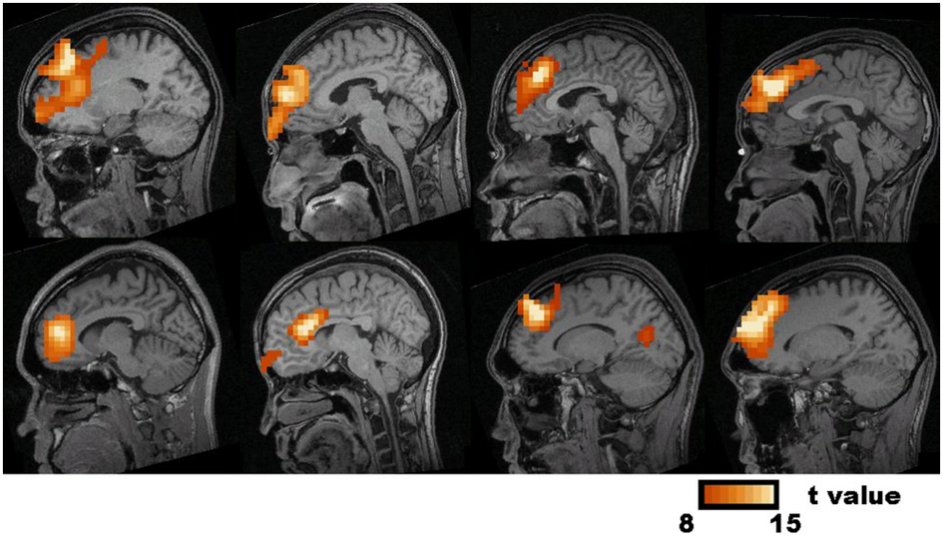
Synthetic Aperture Magnetometry analysis of eight subjects who had prominent medial frontal source power changes in theta frequency band in individual analysis. Statistic parametric maps (SPM) of the Frontal Midline Theta source-current density in individual subjects are projected onto sagittal slices of the subject's MRI. The color bar represents *t*-values.
